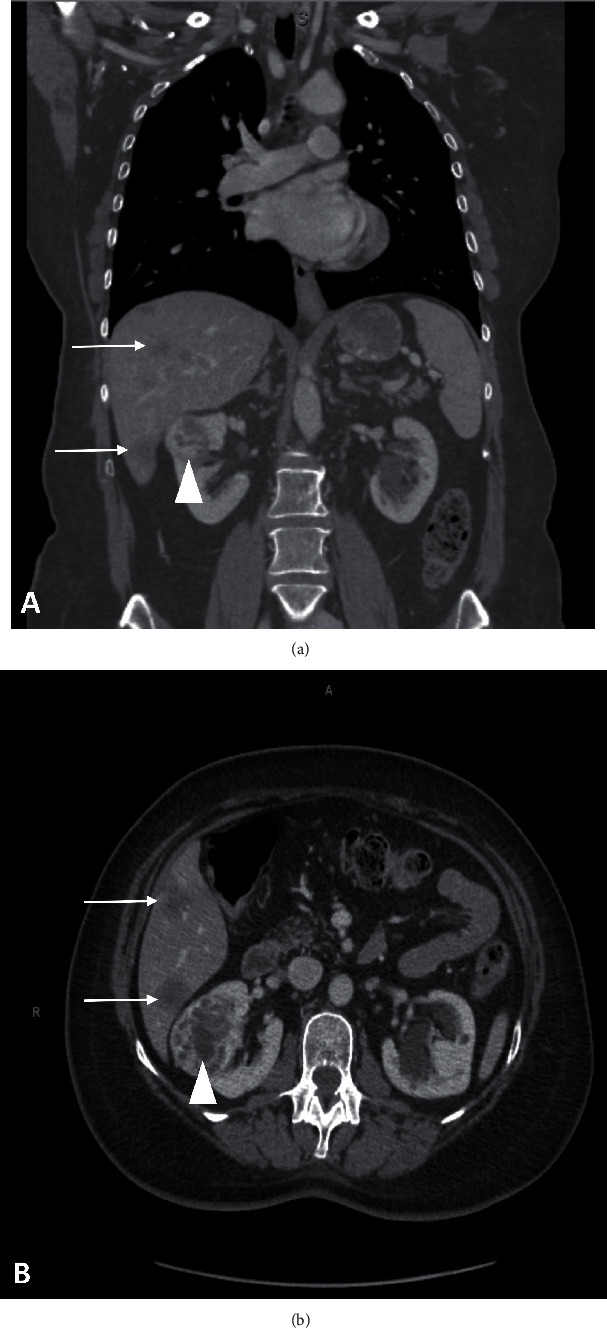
Abdominal CT scan with right renal mass (white arrowheads) and liver metastases (white arrows). (a) Coronal section. (b) Transverse section.
